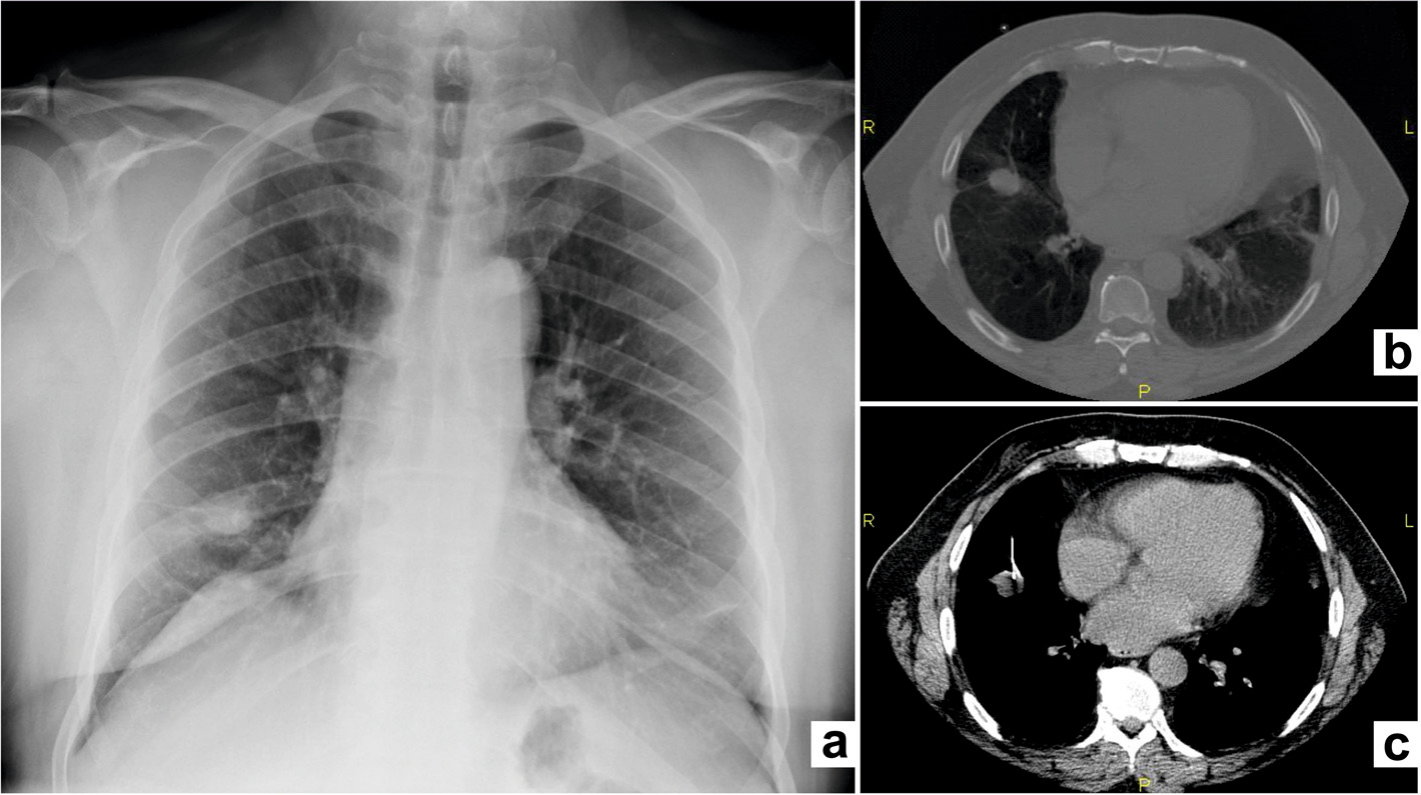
Radiological images of the pulmonary nodule. Chest X-ray showing a lesion in the right lung field (a). Contrast-enhanced computed tomography scan of the chest revealing a rounded, high-density lesion with irregular profiles with a diameter of 24mm in the middle lobe (b). Fine-needle aspiration (c)
